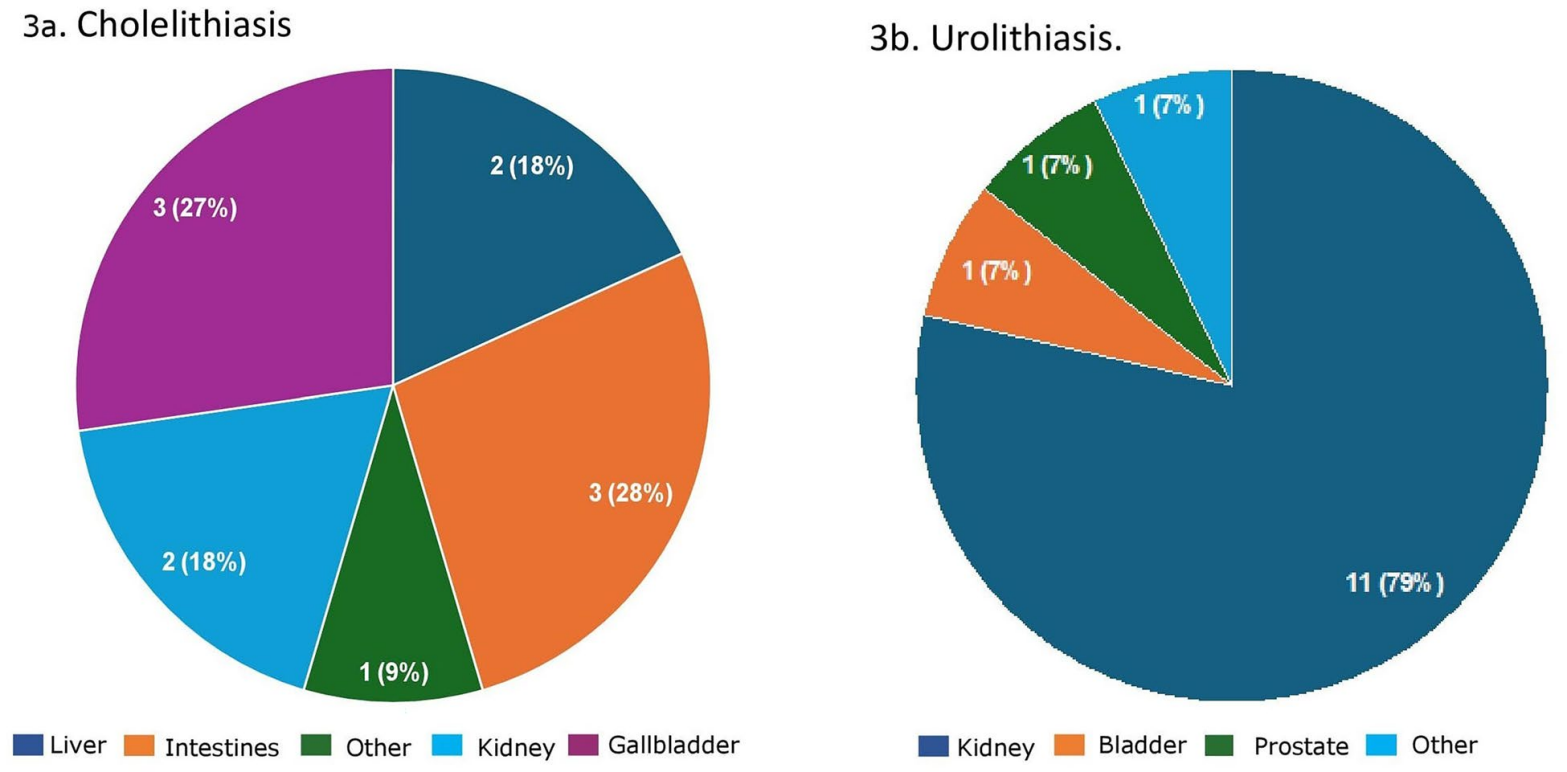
Organ or anatomical structure of the alternative diagnosis for (a) cholelithiasis (*n* = 11), and (b) for urolithiasis (*n* = 14) in reports with only guideline-based indications for abdominal ultrasound.
